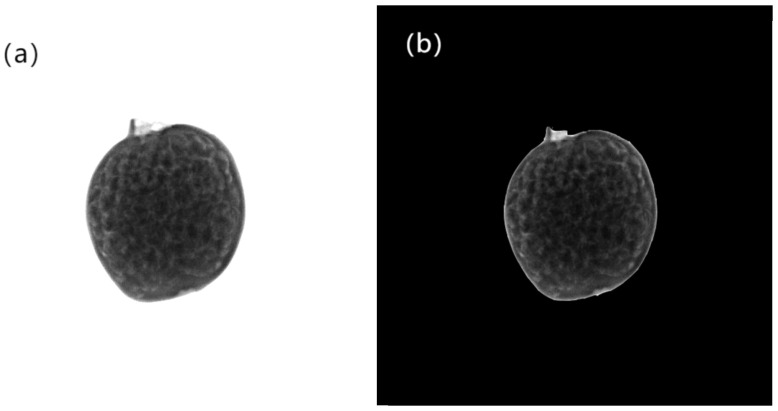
The segmentation process of pomegranate soft X-ray images. (a) Original image. (b) The image after threshold segmentation.
